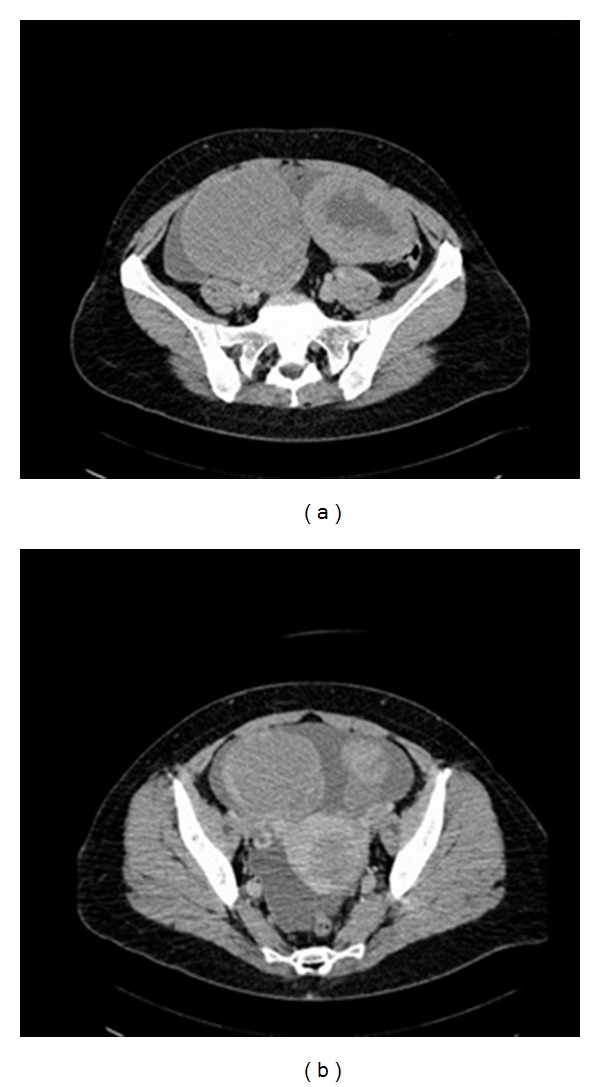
Computed tomography (CT) showed (a) a partially necrotic solid mass measuring 9.5 cm in diameter in the left side of the pelvis and a cystic tumor in right side measuring 10.5 cm. (b) The uterus presented an heterogeneous myometrial structure with a nodular lesion 4 cm long.
